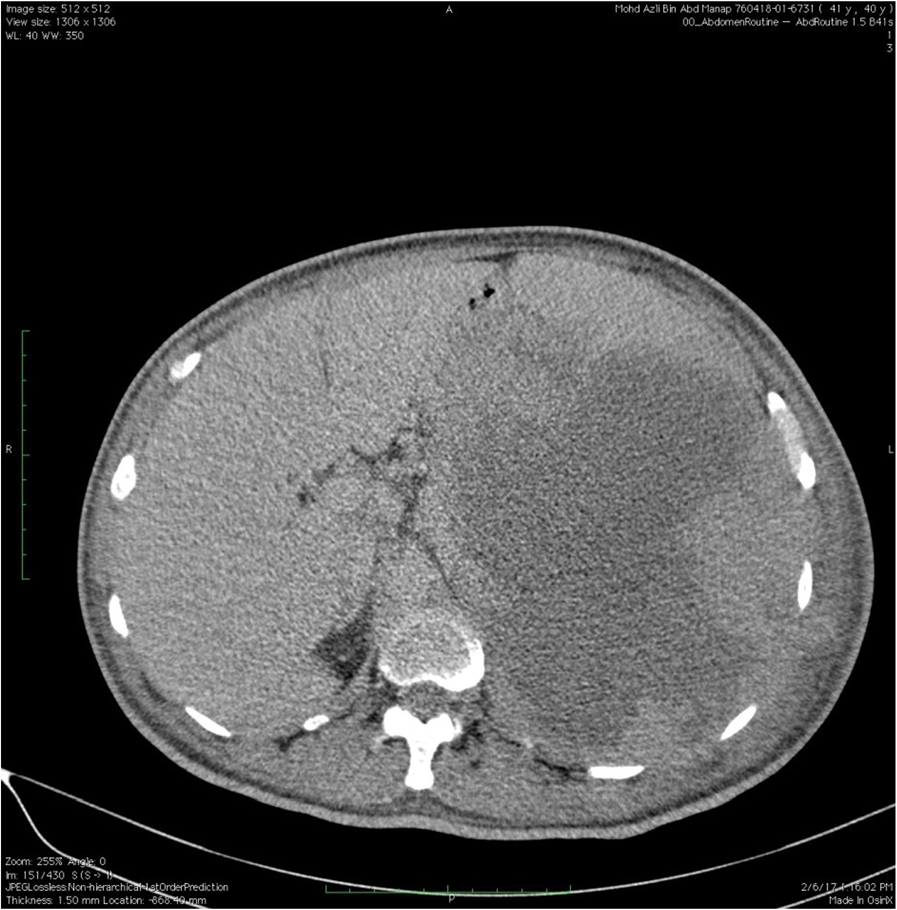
Contrast enhanced Computed Tomography of Thorax, Abdomen and Pelvis showing the abnormal spleen
